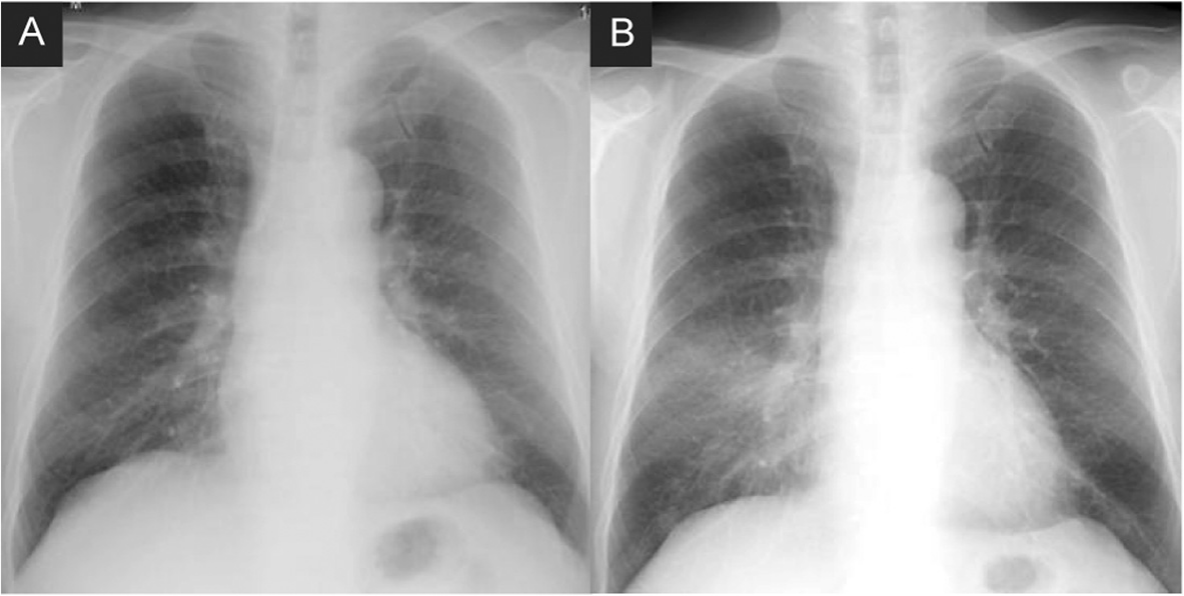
The chest roentgenogram of a 66-year-old male with lipoid pneumonia. No abnormal shadows were detected 6 months prior to admission (a). Infiltrative shadows were noted in the bilateral lower lung fields on admission (b)
